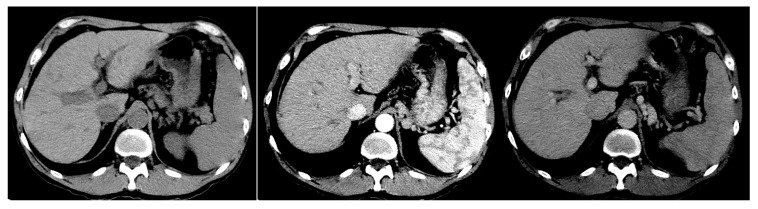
Different CT phased images were obtained from a 52-year-old woman with fibrosis stage F2 due to type C viral hepatitis before and after injection of contrast agent.
